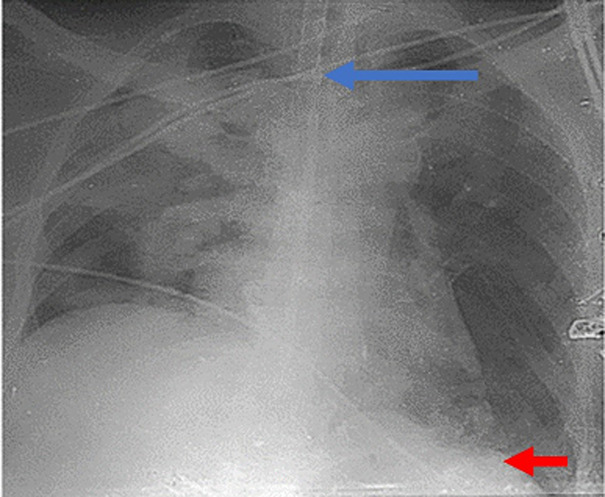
axial and coronal views of the same chest CT scan showing the eventrated left hemidiaphragm (red arrow) and tension viscero-thorax from gastric and colonic subphrenic abdominal components (blue arrow)
